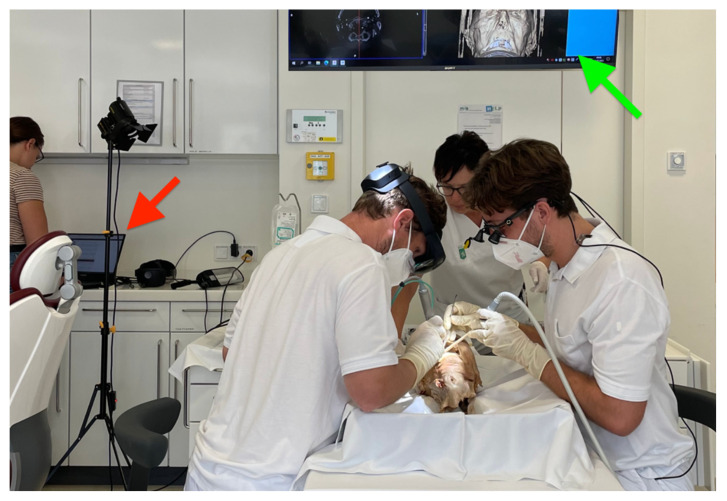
Intraoperative set up at the University Clinic of Dental Medicine and Oral Health, Medical University Graz. The surgeon conducts an apicoectomy on the right maxillary lateral incisor utilizing an augmented reality (AR) image guidance system based on cone beam computed tomography (CBCT) scans. The assistant utilizes illuminated magnifying glasses to optimize lighting during the procedure. A perioperative nurse provides continuous support throughout the surgery. The green arrow indicates the preoperative CBCT scans, while the red arrow marks the technical working station providing the surgeon’s point-of-view. By using the HoloLense 2 as an AR image guidance system, the surgeon’s focus remains completely in the operating field. With this guidance system, the need for the surgeon to alternate attention between the computer workstation and the surgical field is eliminated, allowing complete focus on the operating field by superimposing all image data (including image-based segmentation) into the field of view.
